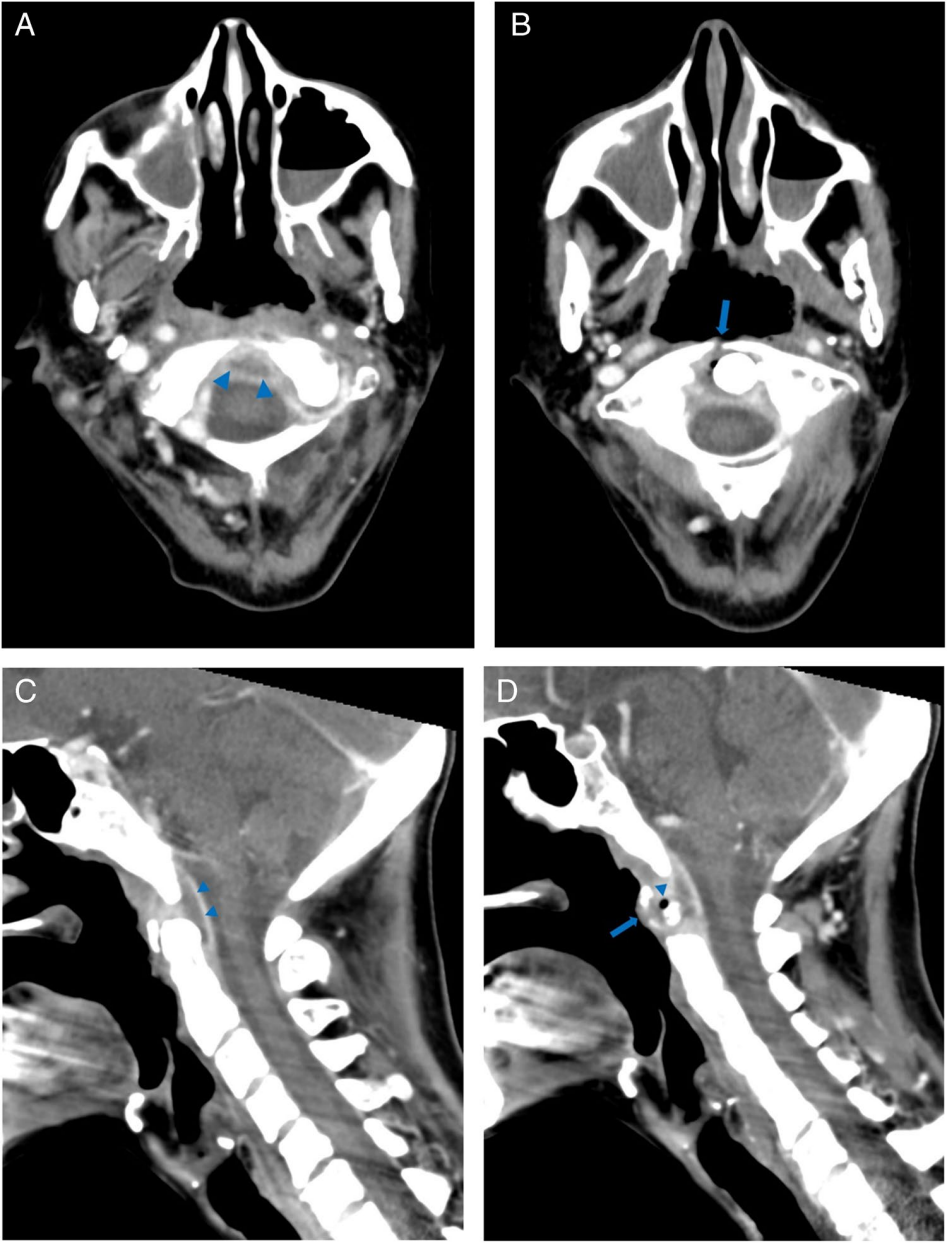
Sequential axial (A and B), midline (C), and paramidline (D) sagittal images from initial contrast-enhanced neck CT demonstrate dehiscence of the posterior oropharyngeal wall with underlying erosion in the C1 anterior arch (arrows) and peripherally enhancing gas-containing fluid collection surrounding the odontoid process (arrowheads)
